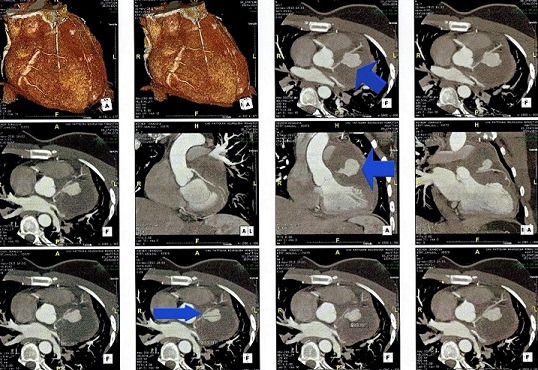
CT angiography identifying a saccular thrombosed aneurysm of the proximal segment of the LAD
